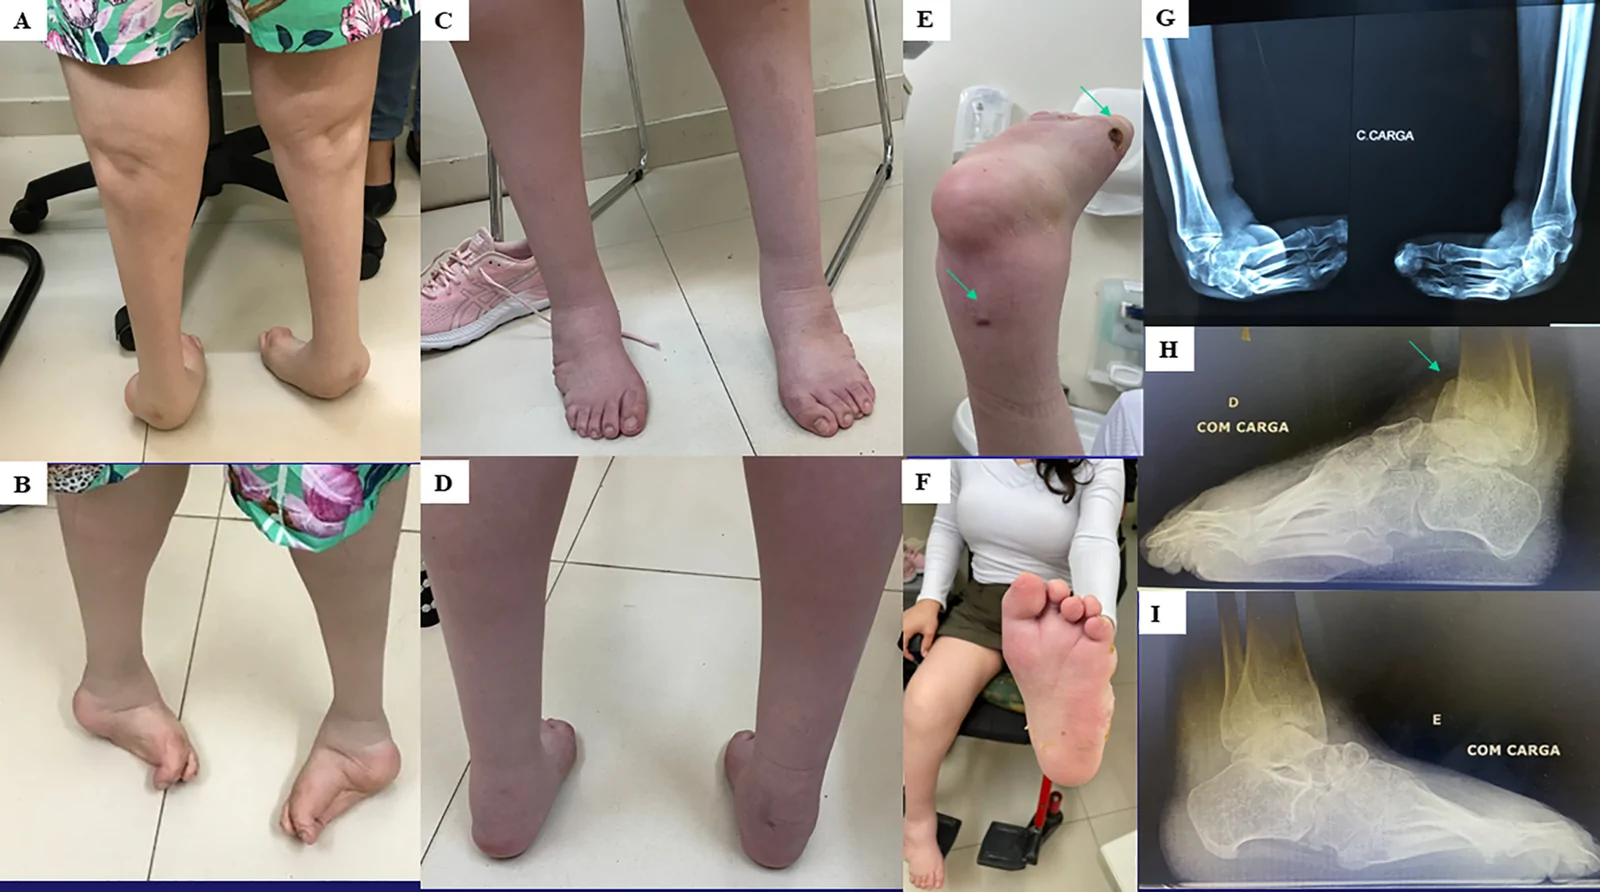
Clinical appearance before treatment. Rigid clubfeet with rigid claw toes in a 38-year-old patient (Patient 3 – Table 3) (A, B). Clinical appearance after treatment. (C, D). The arrow indicates the tenotomy scar (E). The patient has plantigrade feet. Radiographs before treatment (G). Radiographs after treatment. In the right foot, there was a fracture of the distal anterior tibia after the dorsiflexion maneuver to correct the equinus (H, I).
